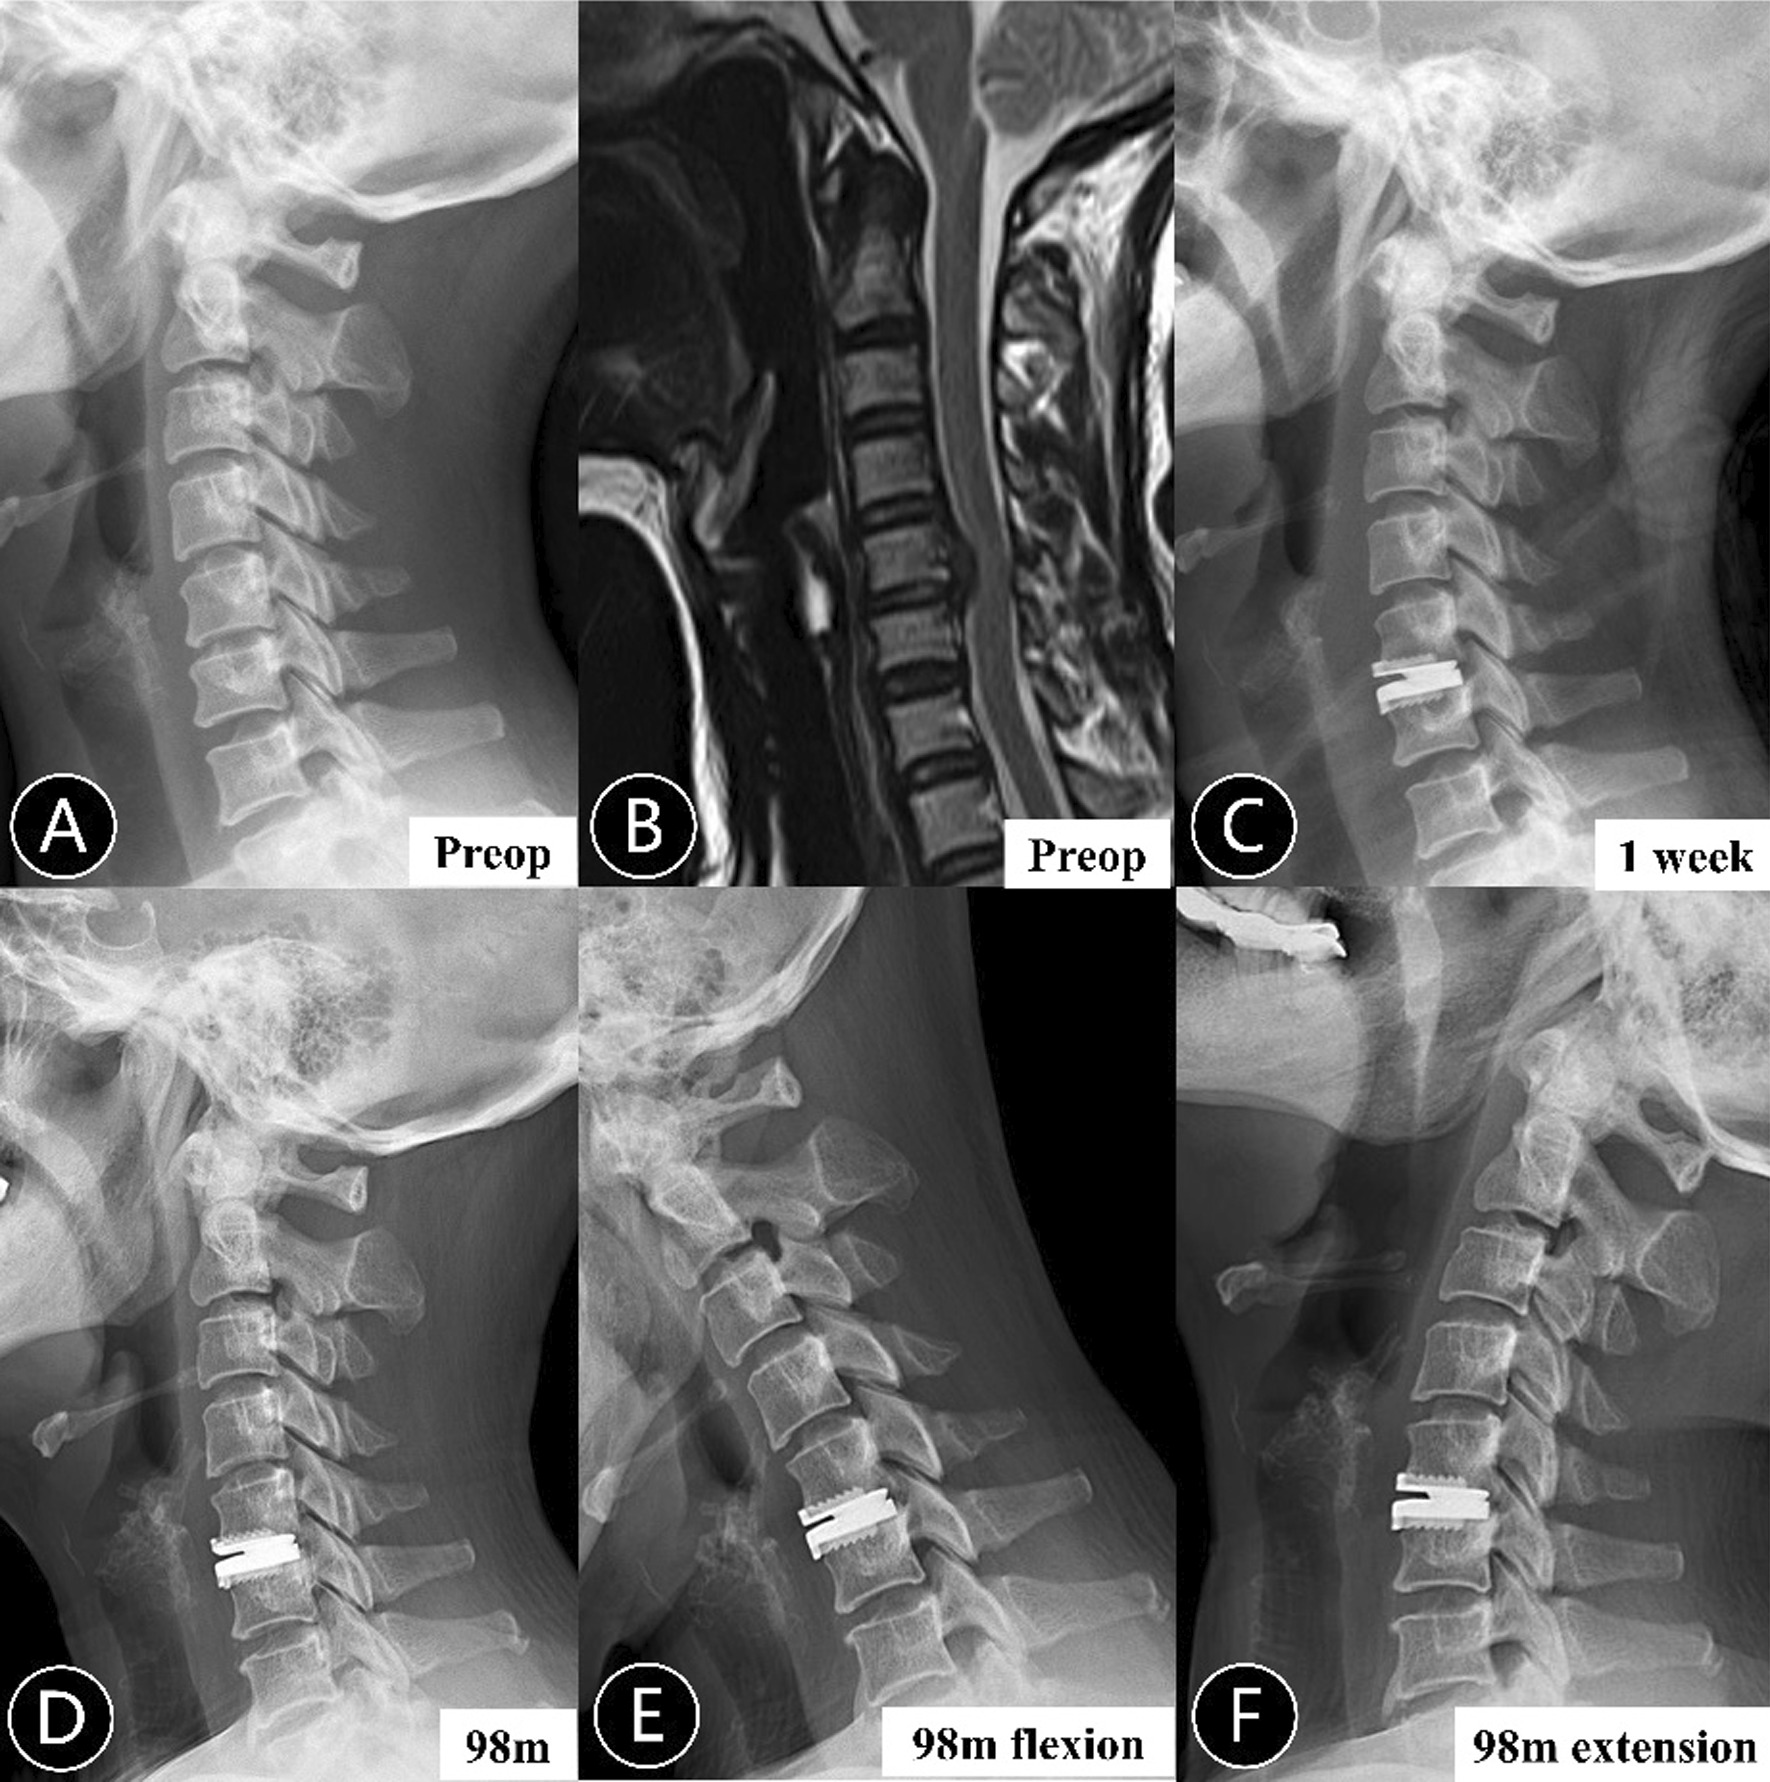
Radiograph of a 50-year-old woman. A, B Preoperative lateral radiograph and MRI showed decreased intervertebral disc height and compression at C5/6. C Lateral radiograph at 1 week after surgery showed a recovery of intervertebral disc height and insufficient endplate coverage at the index level. D–F X-rays at 98 months follow-up suggested posterior heterotopic ossification with motion preservation at C5/6
